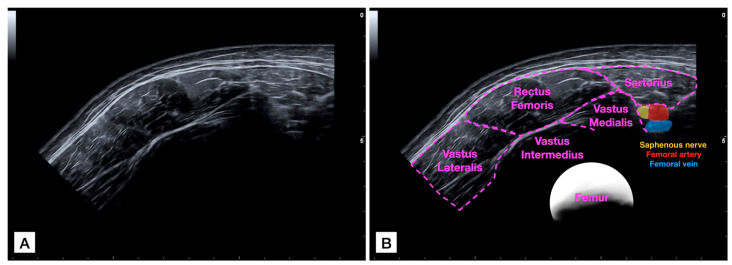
Raw panoramic ultrasound (US) imaging acquisition of the rectus femoris muscle (A) and image with identification of structures (B).
